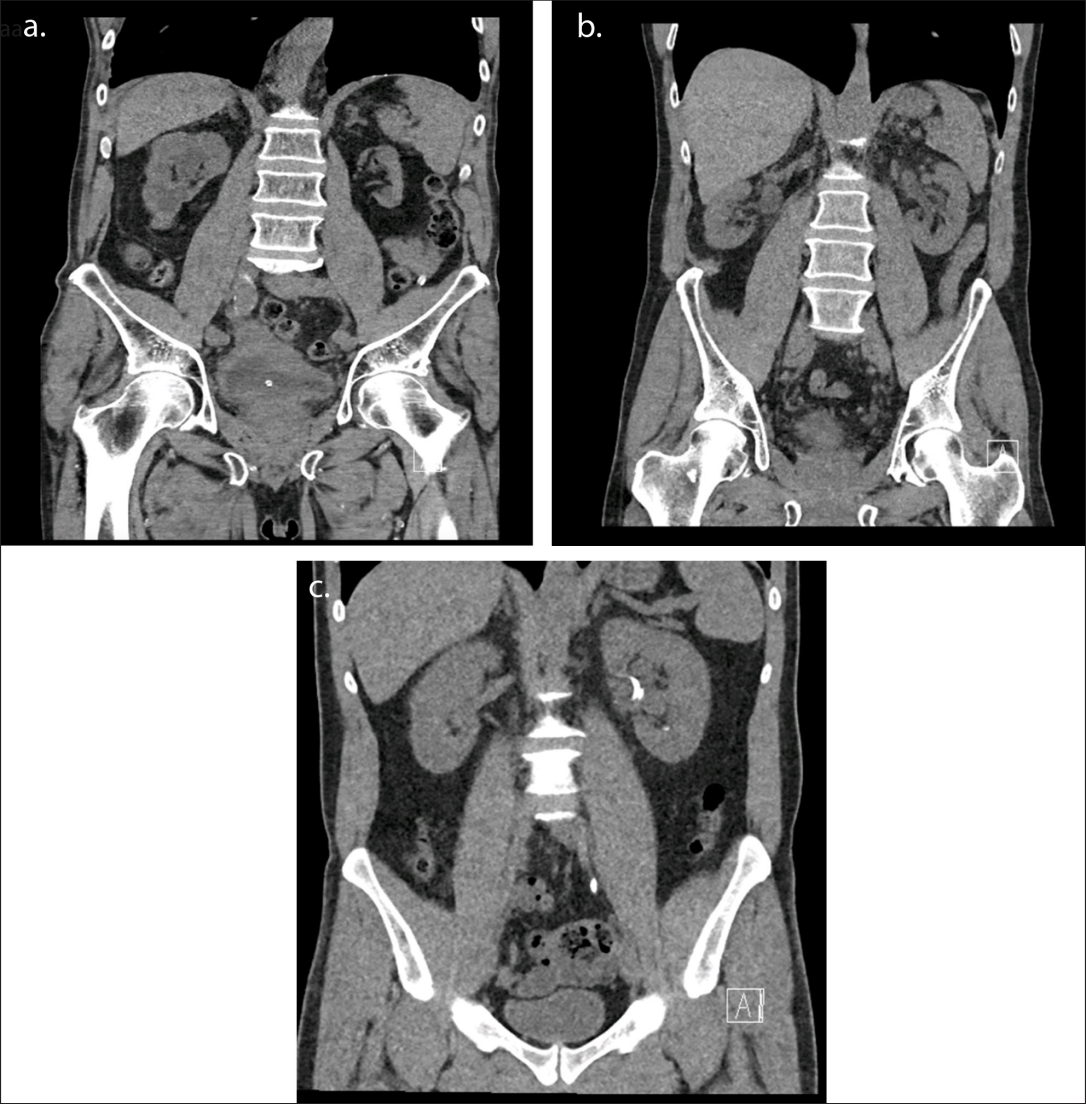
CT examination for urolithiasis in coronal section for three patients of similar diameters: (a) wrong acquisition length and protocol selection – DLP 206.1 mGy.cm (b) right protocol but wrong acquisition length – DLP 82.4 mGy.cm (c) right protocol selection using Tin Filter and right acquisition length – DLP 34.3 mGy.cm.
